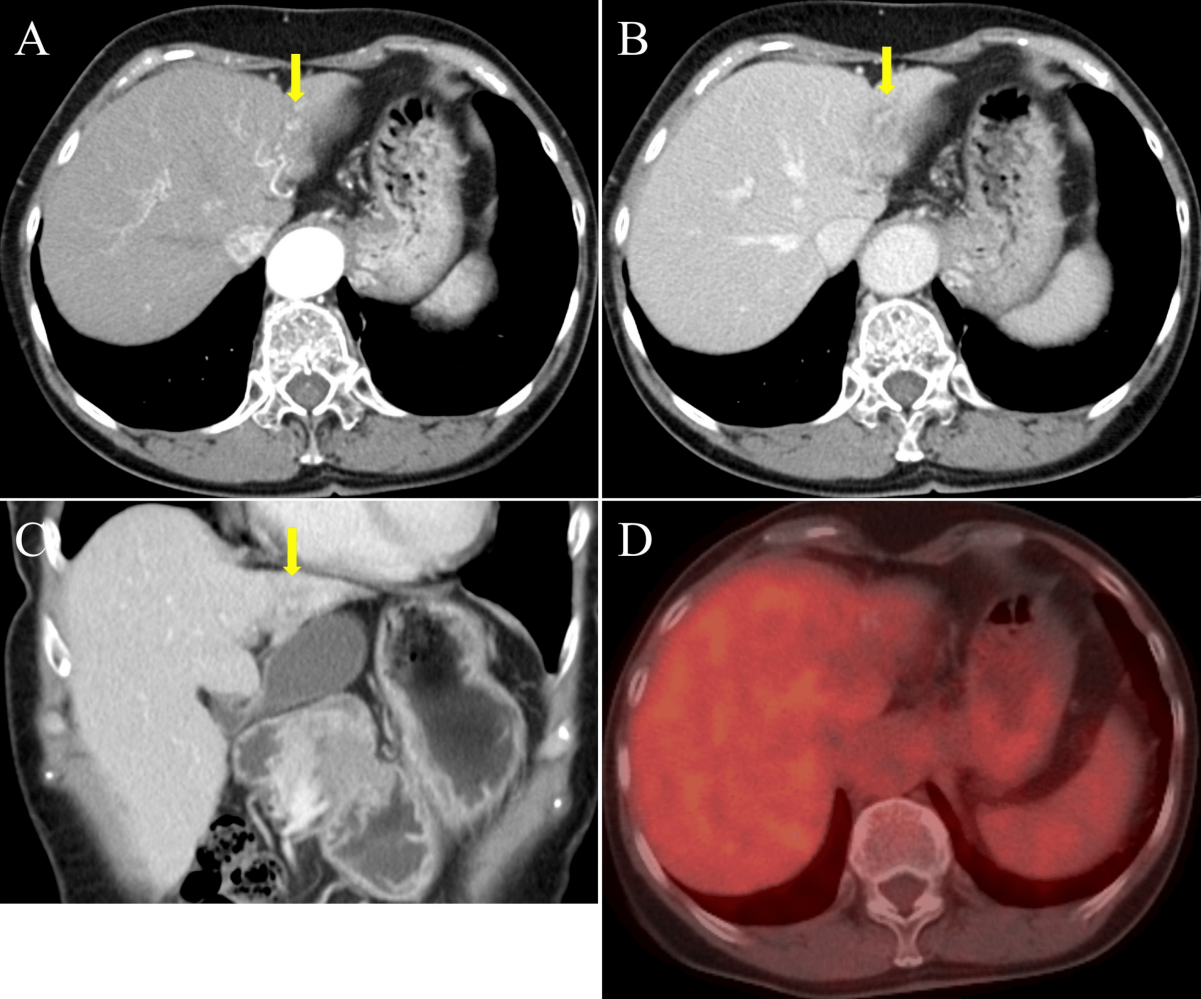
CT images 18 months after HCC diagnosis A-C: Follow-up CT images showed further regression of the left lobe, with a decrease in the size of the residual low-attenuation area in the lateral segment (shown by the yellow arrow). D: 18F-Fluorodeoxyglucose (FDG) positron emission tomography/CT showed regression of the previously observed hypermetabolic tumors, with no pathological FDG uptake in the liver. HCC: Hepatocellular carcinoma
